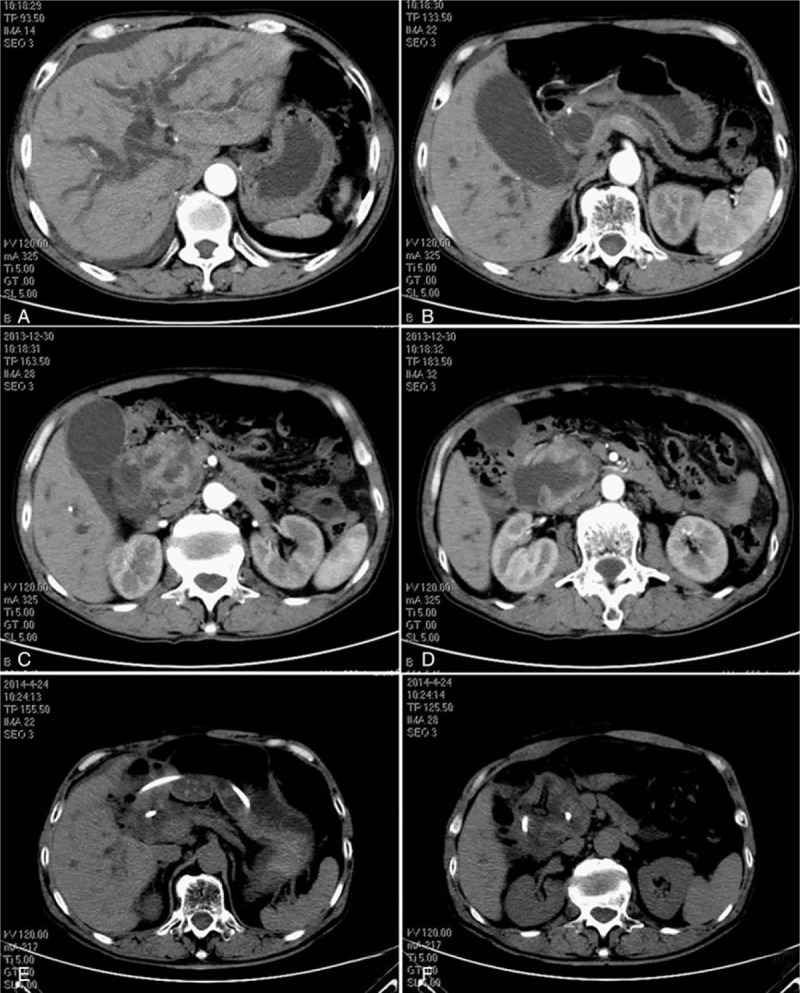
(A and B) Contrast-enhanced CT scan showed marked dilatation of intrahepatic and extrahepatic bile ducts in the absence of any luminal filling defect, main pancreatic duct of size >10 mm and signs of distal pancreatic atrophy. (C and D) A heterogeneous, cystic mass measuring 4.2 × 5.4 cm involving the duodenum and pancreatic head with thickened enhanced cyst walls, with an abnormal communication between the mass and the descending part of the duodenum. (E and F) Obstruction of biliary and pancreatic duct was significantly decreased after associating pancreaticostomy and biliary-irrigation.
